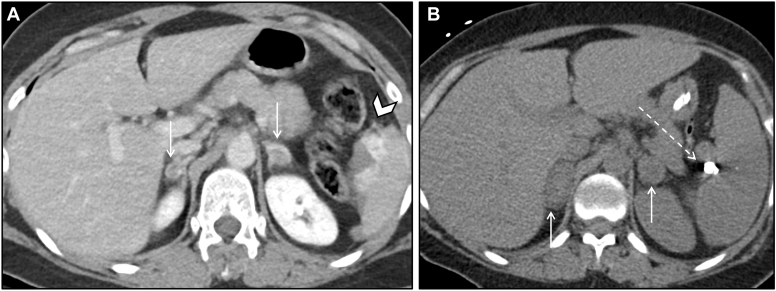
Bilateral adrenal hemorrhage due to trauma. Axial contrast-enhanced CT in the portal venous phase (*A*) demonstrates bilateral adrenal hyperattenuating lesions (arrows) that were new from a prior CT scan done 9 months ago. These enlarged on subsequent CT performed 12 days later (*B*), consistent with bilateral adrenal hemorrhage. Initial CT additionally shows active bleeding from a splenic laceration (arrowhead), with splenic artery embolization coils noted on subsequent CT (dashed arrow). *CT* = computed tomography.
